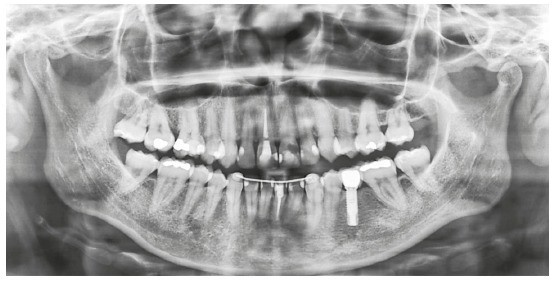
Panoramic radiograph of 10-years post-retention follow-up.
